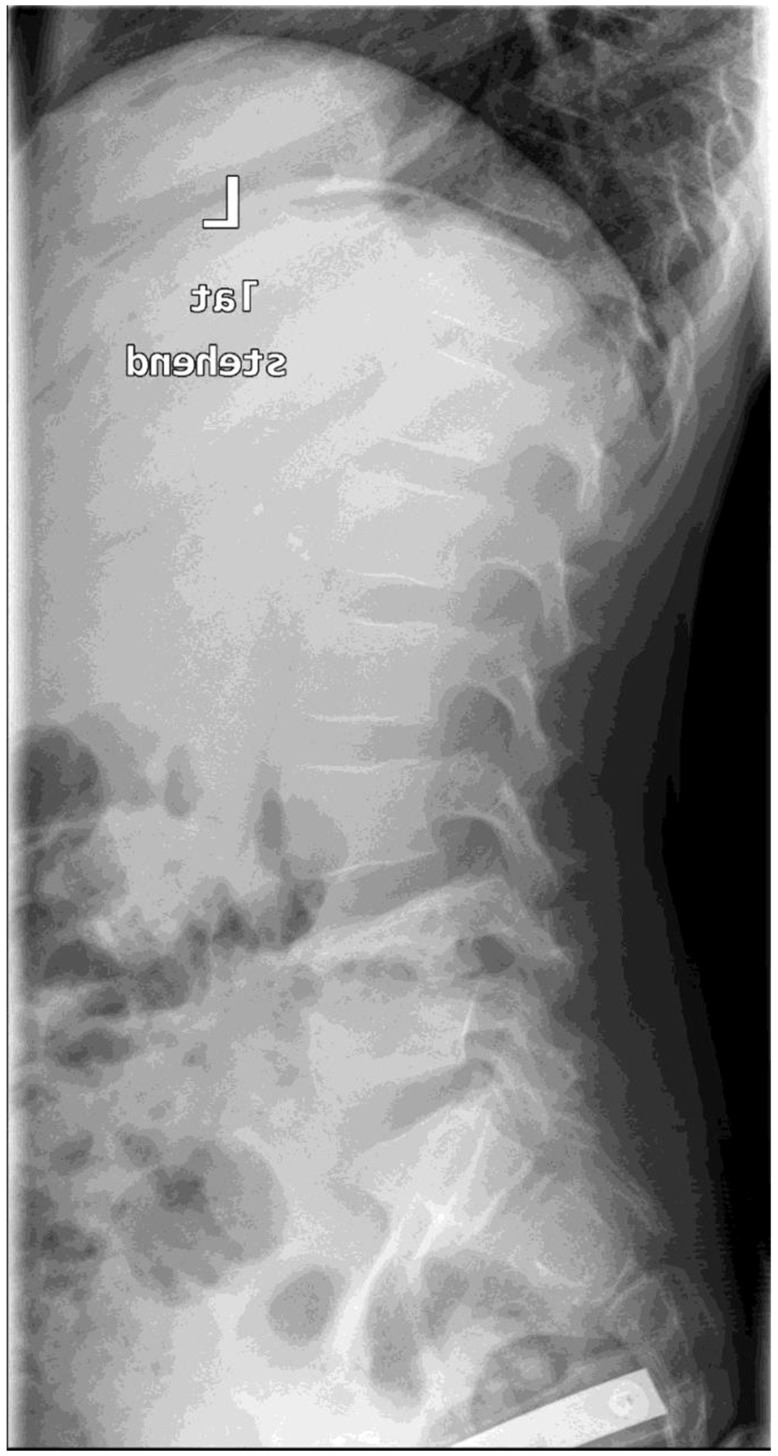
X-ray (sagittal view) of the lumbar spine (Pat. No. 10).
